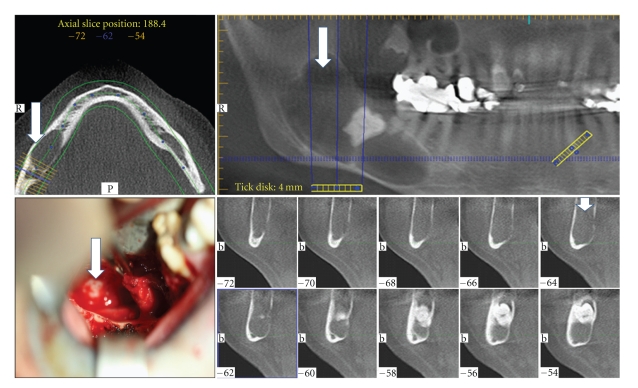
Mandibular defect following cyst. CT scan of huge cyst in the mandible (see white arrows). The clinical picture represents the situation after removing the cyst revealing the alveolar nerve positioned at the bottom of the cavity (black arrow).
